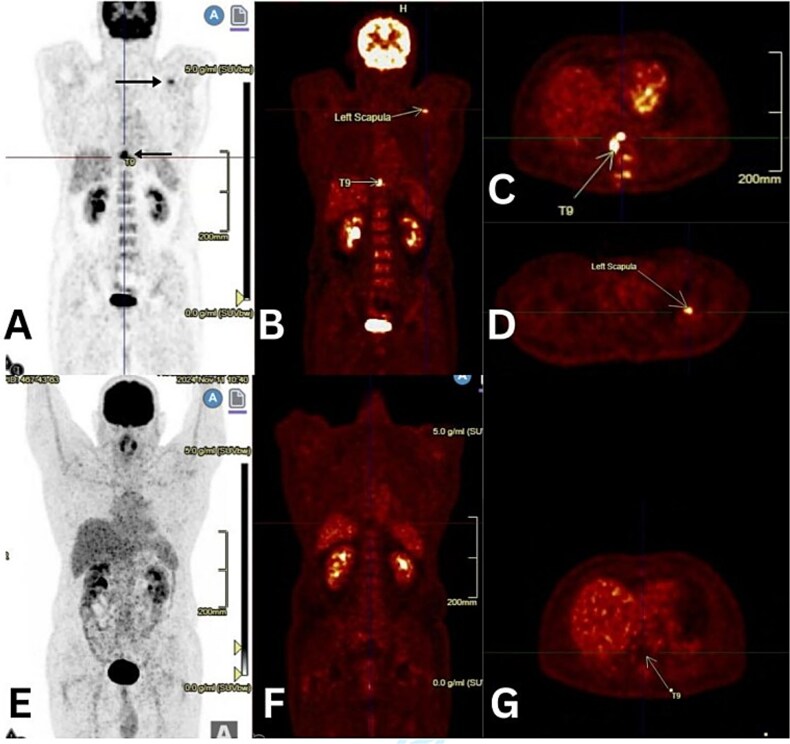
Figure 4 (A-D) initial PET/CT images showing abnormal uptake at the T9 vertebra and left scapula (black and yellow arrows). (E-G) end-of-treatment PET/CT images showing resolution of abnormal uptake.
